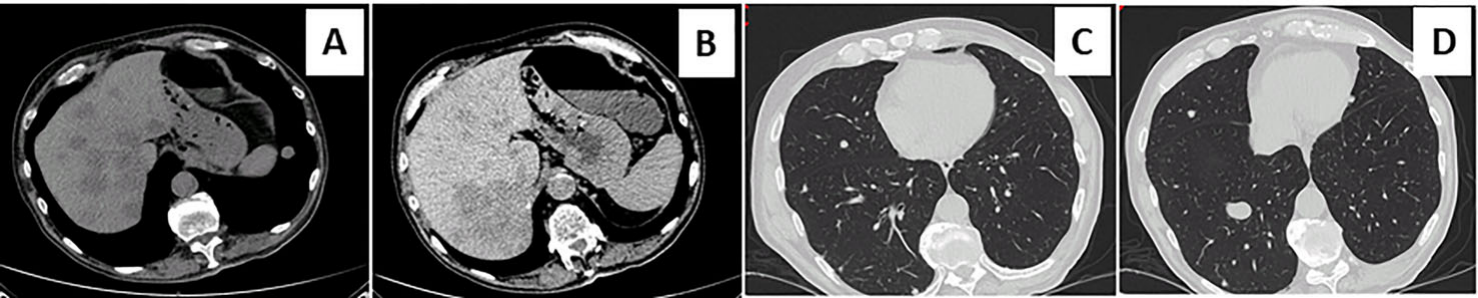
Metastatic diffusion at chest and hepatic computed tomography scan. Contrast-enhanced abdominal and chest computed tomography scan showed the appearance of multiple metastatic pulmonary and hepatic nodules (A–D).
